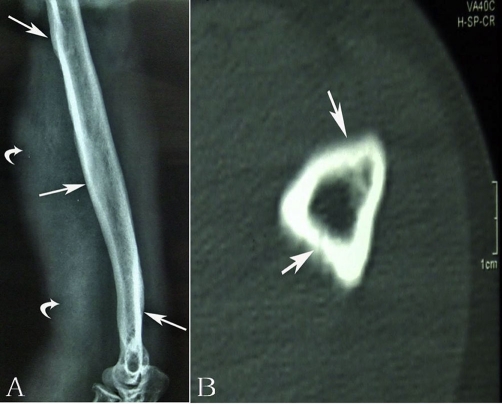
(A) Radiograph of left humerus revealed extensive cortical thickening and prominent sclerosis (*long arrow*) were presented along the humeral shaft. Swellon soft-tissue mass (*curved arrow*) was also visible. (B) CT image demonstrated extensive periosteal reaction and cortical osteolysis were presented along the proximal humerus. The medial aspect of humerus showed irregular osteolysis with pathologic fractures. (*white arrow*).
